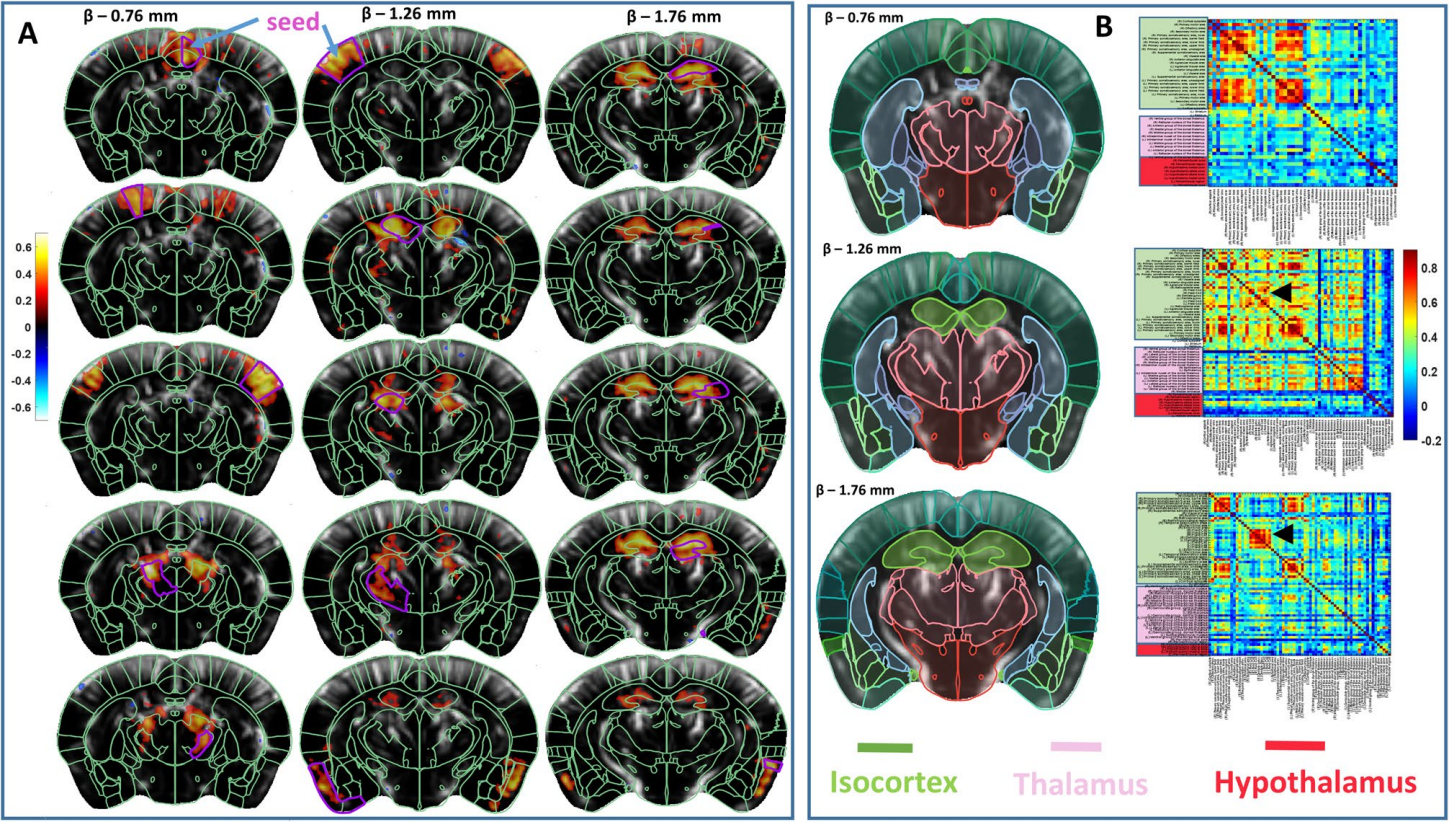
Transcranial functional connectivity analysis with BPS and atlas-based segmentation. (A) Seed-based analysis. The grayscale images represent the baseline Power Doppler images. Seed regions are indicated in magenta. Color-coded correlation maps are overlaid to baseline images for each of the selected seed regions. Automatically aligned anatomic delineations from the Allen CCF are shown in green for reference. (B) Connectivity matrix analysis. BPS enabled anatomic boundaries delineation and data extraction from about 70 regions for each of the 3 imaging planes. Regions are overlaid onto the baseline Power Doppler images. Connectivity matrices were computed based on pairwise correlations between signals from individual regions. The matrices show color-coded correlation coefficients. Arrowheads in (B) indicate connections within the hippocampal formation.
